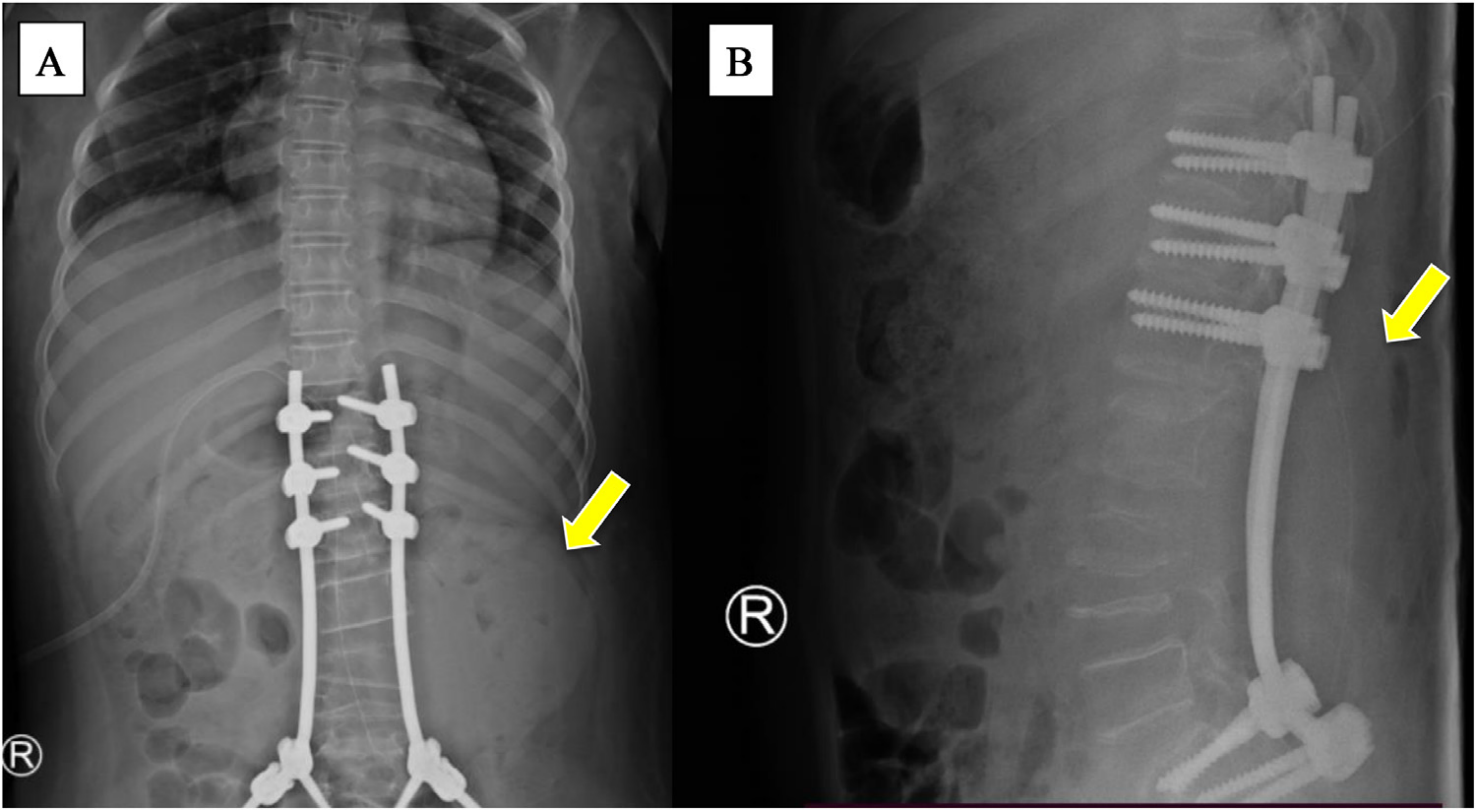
Post percutaneous sclerotherapy x-ray: AP view (A), and lateral view (B) showed the mass size was reduced.
